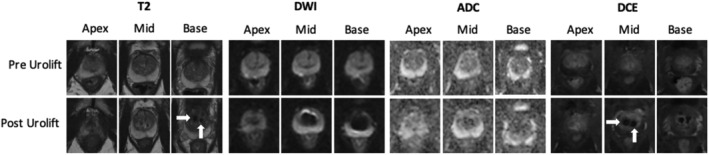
Multiparametic Magnetic Resonance Imaging Pre and Post PUL insertion. Artifact can be appreciated particularly in the T2 phase and DCE phases as dark areas (white arrows). [Correction added on 19 June 2024, after first online publication: Figure 2 caption has been corrected.]
